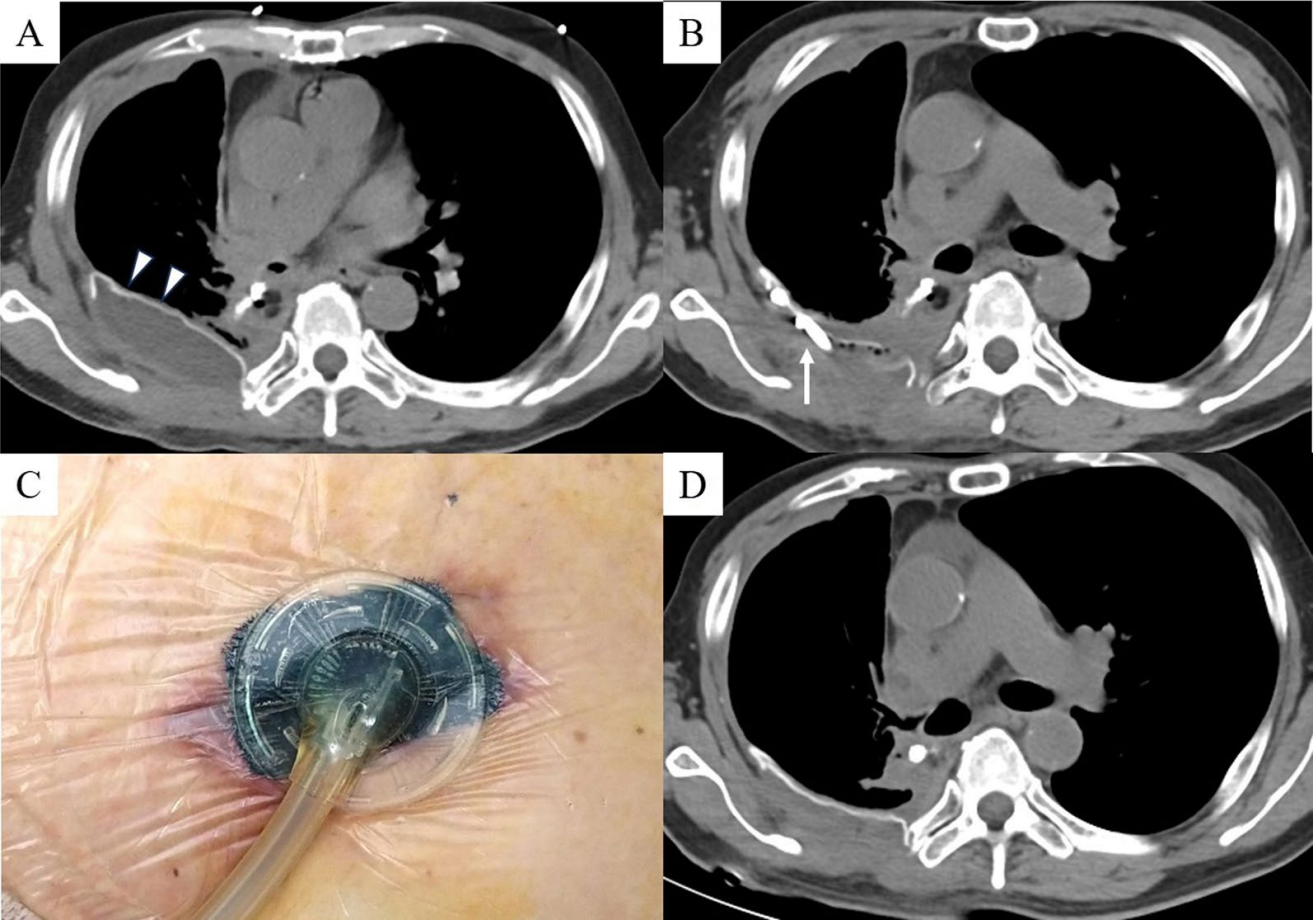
A Chest computed tomography (CT) showed fluid collection in the chest wall around chest wall reconstruction by expanded polytetrafluoroethylene (PTFE) sheet (white arrowheads). B The fluid collection in the chest wall was decreased by continuous drainage (white arrow). C Vacuum-assisted closure (VAC) therapy was performed for chest wall infection. D The space around the PTFE sheet had decreased on chest CT after VAC therapy
